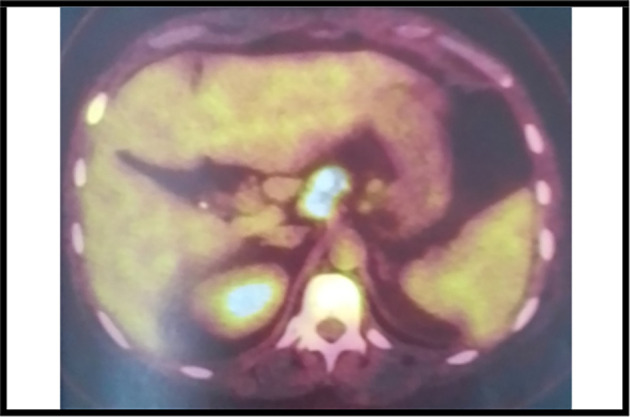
A PET scan image showing the subphrenicganglionar fixation of the FDG.
